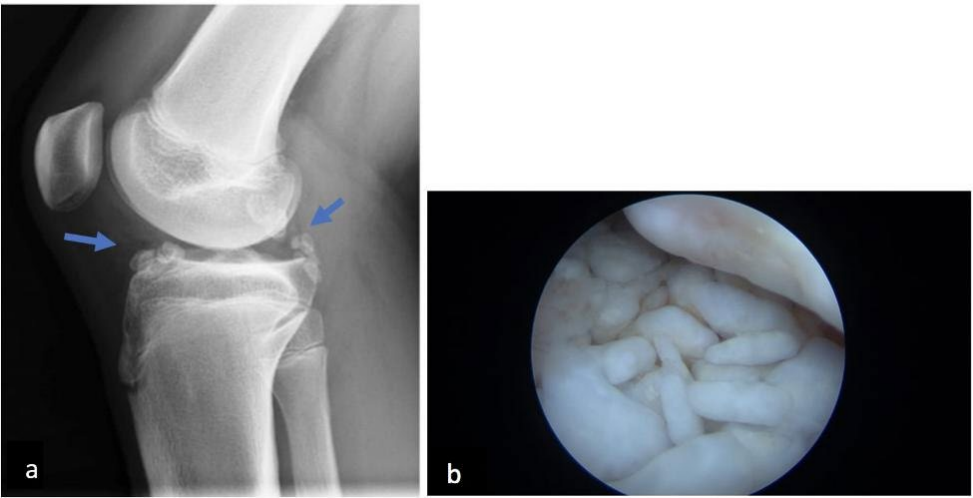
X-Ray of the right knee: multiple radiopaque, round, loose bodies within the joint (a). Arthroscopy during synovectomy: multiple white, shiny loose bodies were within the articular space (b)
